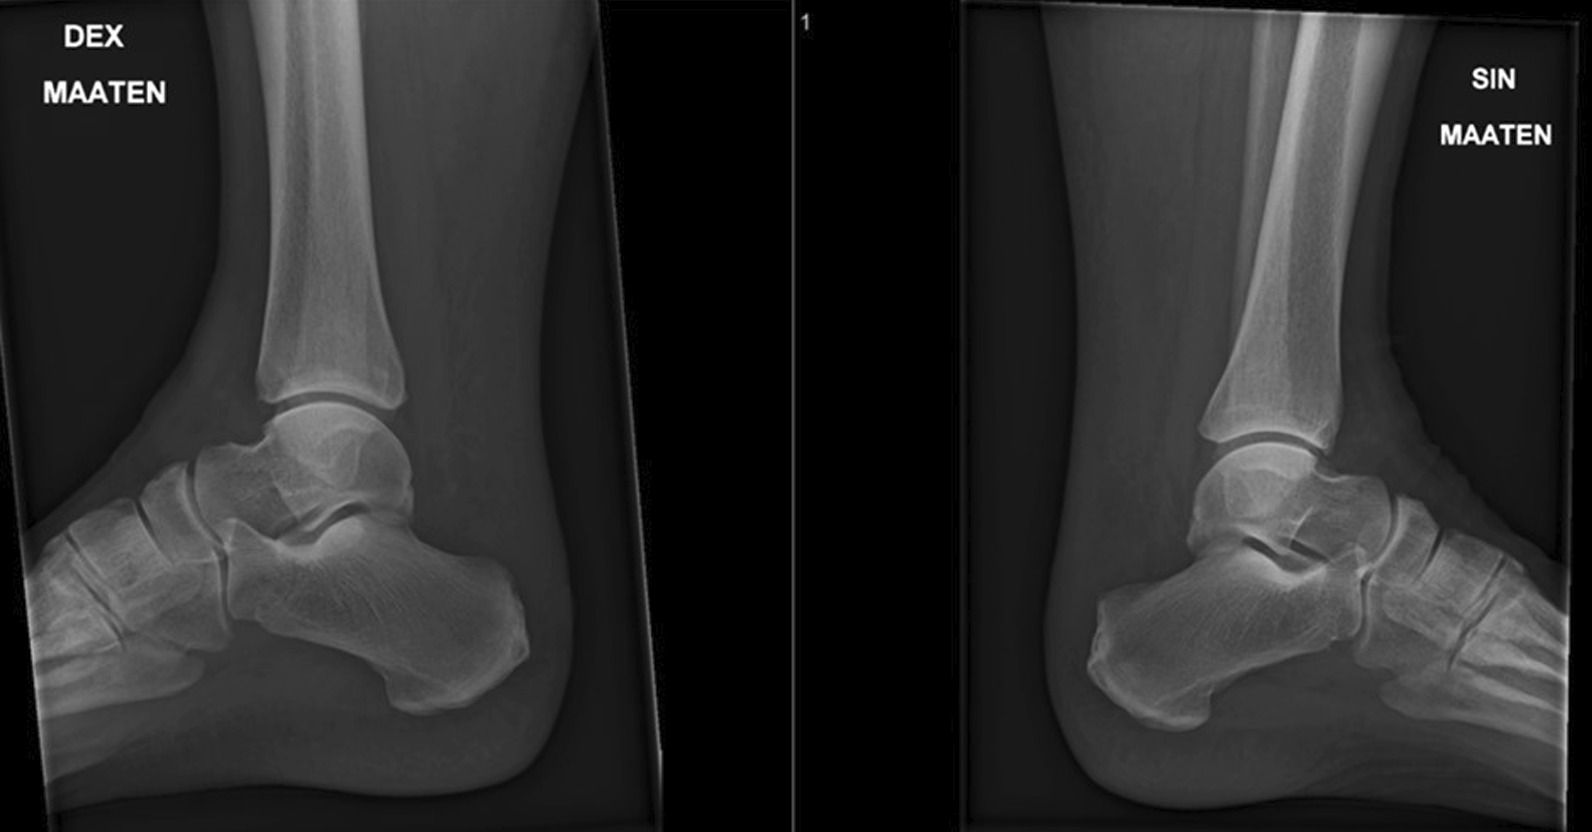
X-rays showing no bony traumas on either side
